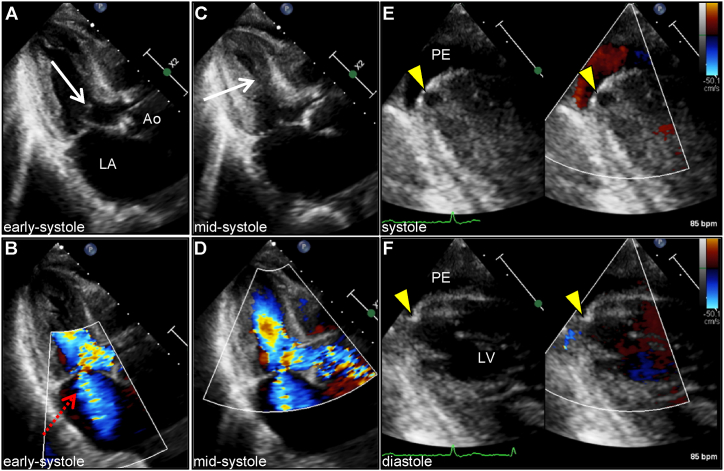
Transthoracic echocardiography. A transthoracic echocardiography reveals mild left ventricular hypertrophy (11 mm) and reduced chamber size (38 mm) with normal contraction (ejection fraction of 64%). Parasternal long-axis view reveals a sigmoid-shaped interventricular septum and an elongated anterior mitral leaflet with systolic anterior movement (arrow), resulting in septal contact leading to dynamic left ventricular outflow tract obstruction at early systole (A), concomitant with significant secondary mitral regurgitation with a posterior jet (dashed arrow) on color flow Doppler (B). It is notable that mid-cavity obstruction was observed (arrow) at mid-systole (C). Color Doppler echocardiography shows a peak flow velocity of 3.7 m/s at mid-systole, corresponding to a pressure gradient of 54 mmHg between the apex and basal of the LV (D). The modified apical four-chamber view shows an outpouching of the left ventricular apex that is remarkably visible during systole (arrowhead) and a moderate amount of pericardial effusion (E, F). The apparent flow is not recognized in apical outpouching by color Doppler. Ao, aorta; LA, left atrium; LV, left ventricle; PE, pericardial effusion. (For interpretation of the references to color in this figure legend, the reader is referred to the Web version of this article.)
